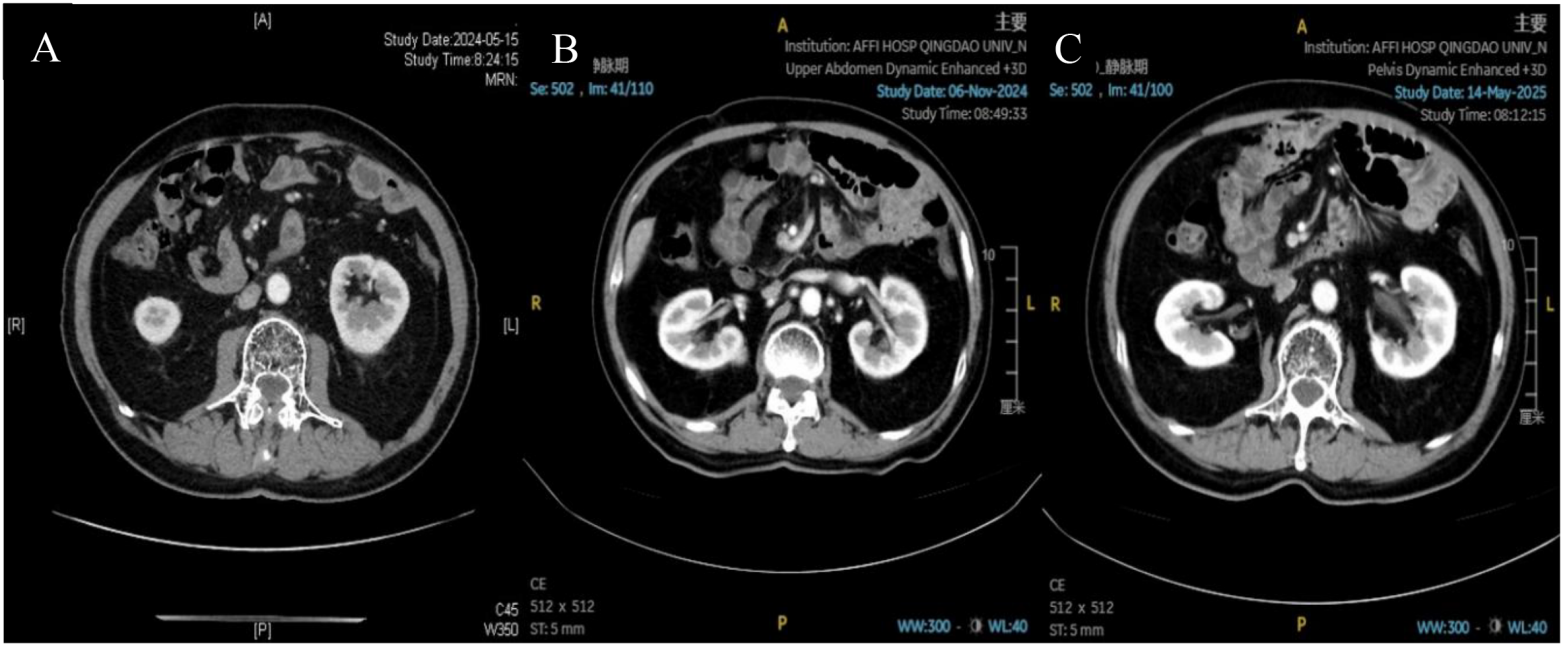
Surveillance abdominal CT scans. Images from May 2024 (A), November 2024 (B), and May 2025 (C) show stable post-treatment changes and no evidence of recurrent disease.
